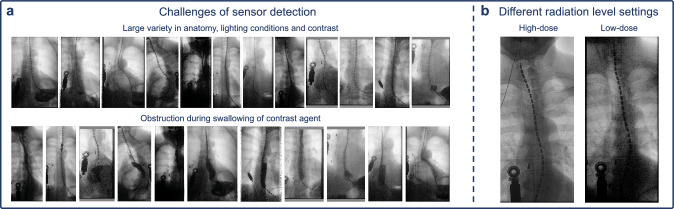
Examples showing the challenges of the sensor detection in the X-ray images (a), as well as a comparison between a high-dose and a low-dose X-ray image of the same patient (b)
